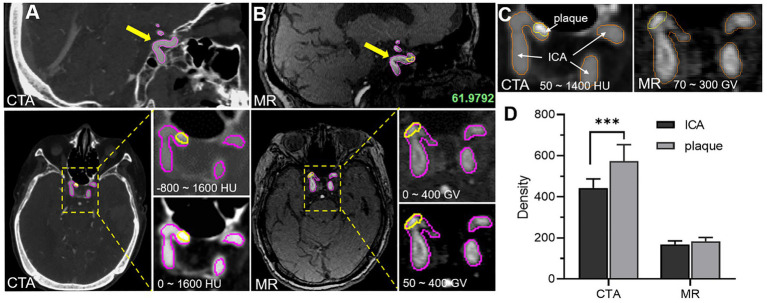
Comparative analysis of image characteristics of CTA and TOF-MRA (A) CTA images of carotid artery and plaque, and yellow and magenta circle showed the plaque area and carotid artery area separately (B) MR images of carotid artery and plaque. (C) The density of carotid artery and plaque density by the two methods. (D) The statistical difference of carotid artery and plaque density between the two methods.
